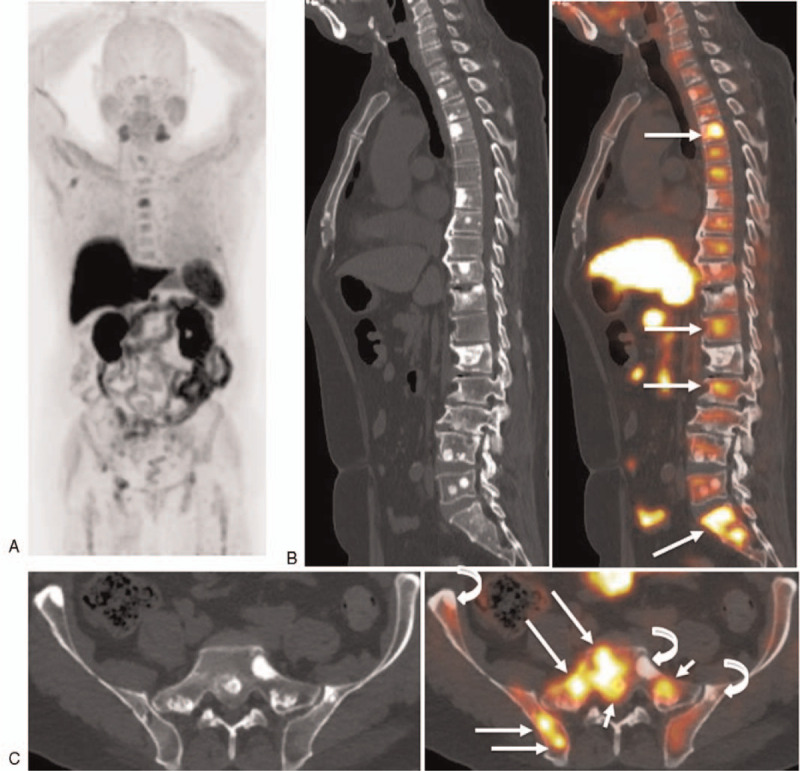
Seventy-five-year-old man with PSA level of 13.41 ng/mL who underwent hormonal therapy and docetaxel for prostate cancer (cT3N0M1b, initial PSA 81.2 ng/mL, Gleason score 5 + 5), in whom choline PET/CT could clearly detect viable progressive osteoblastic bone metastasis, benign osteoblastic change induced by the treatment effect and viable non-osteoblastic bone metastasis. (A) MIP from ^11^C-choline PET/CT image shows abnormal ^11^C-choline uptakes in the spine and pelvic bone. (B) Sagittal ^11^C-choline PET/CT image shows multiple osteoblastic lesions in the spine with no ^11^C-choline uptakes, reflecting treatment-induced sclerosis during the treatment course and several abnormal ^11^C-choline uptakes in the thoracic (Th4,12) lumbar spine (L2), and sacral (S1) spine non-sclerosis (long arrows), suggesting non-osteoblastic bone metastasis. (C) Axial ^11^C-choline PET/CT shows 2 osteoblastic lesions in the sacral spine (S1) and left ilium with no ^11^C-choline uptakes (curved arrow), reflecting treatment-induced sclerosis during the treatment course, 2 abnormal ^11^C-choline uptakes in the S1 sclerosis (short arrows), suggesting viable tumors, and abnormal ^11^C-choline uptakes in the non-osteoblastic lesions of the S1 and right ilium (long arrows), reflecting viable non-osteoblastic bone metastasis.
